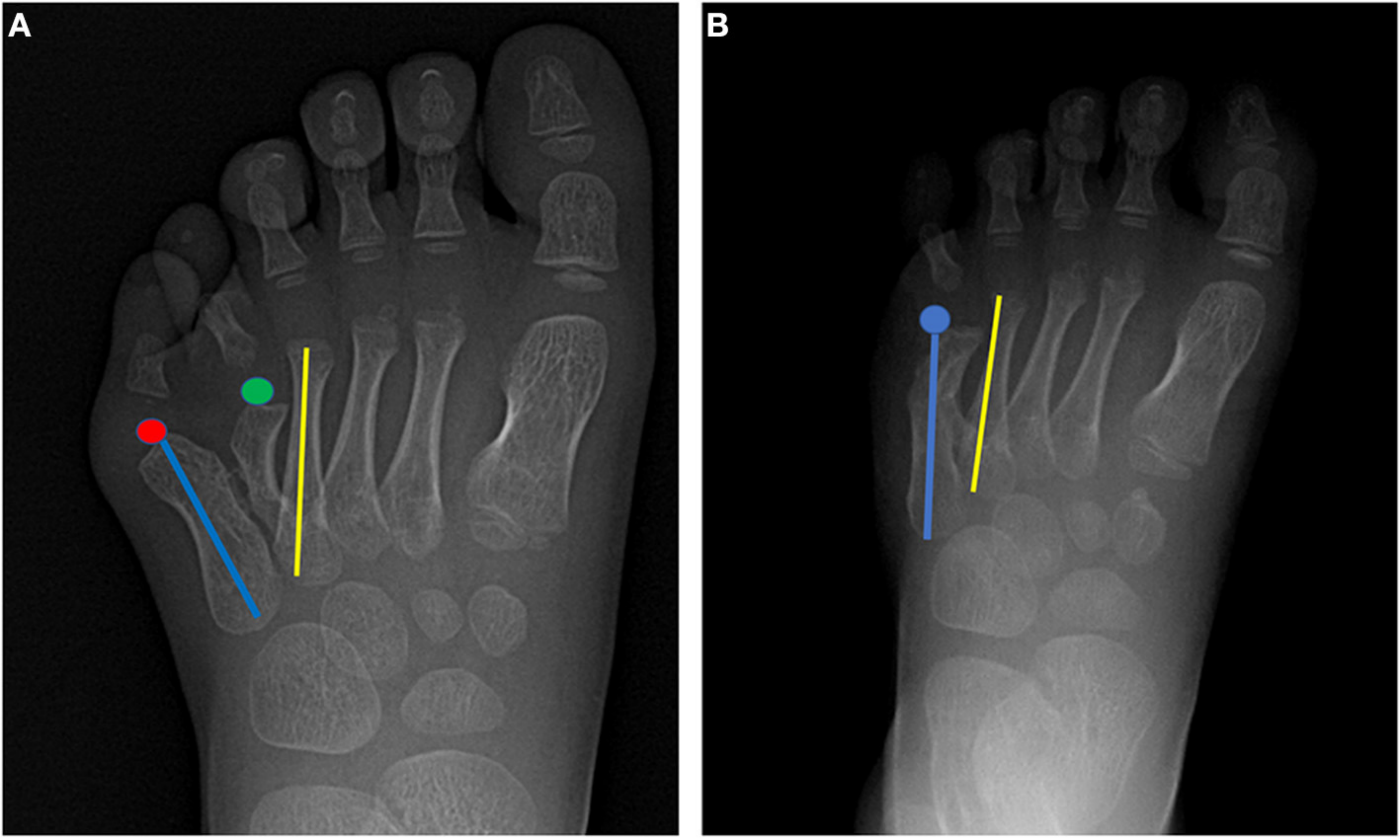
Pre- and postoperative radiographs for comparison of foot morphology. (A) The red dots represent the centers of the lateral metatarsal bones, and the green dots represent the centers of the medial metatarsal bones. The blue line represents the axis of the fifth metatarsal, and the yellow line represents the axis of the fourth metatarsal. (B) The blue line represents the axis of the fifth metatarsal bone after surgery. The blue dot represents the center of the fifth metatarsal bone after surgery; the sequence of the fifth toe line returned to normal after surgery.
